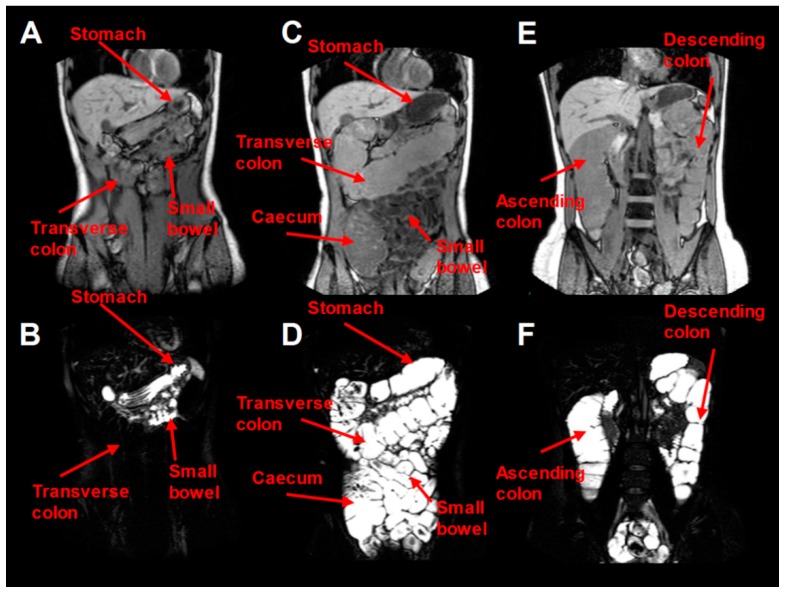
MRI quantification of water colonic content of one volunteer after ingestion of a single 2 L PEG electrolyte scheme. (A,B) concern fasting baseline conditions. White colour stands for water presence and black colour for water absence. In (B), water was found in the small bowel and the stomach (limited resting fluid) but not in the transverse colon. (C–F) conditions appear immediately after the ingestion of the 2 L PEG dose and therefore in (D) water was found in the stomach, the cecum and the transverse colon while (F) is a posterior image and represents water appearance in the ascending and descending colon. Reproduced from [25], Blackwell Publishing LTD, 2014 with permission.
